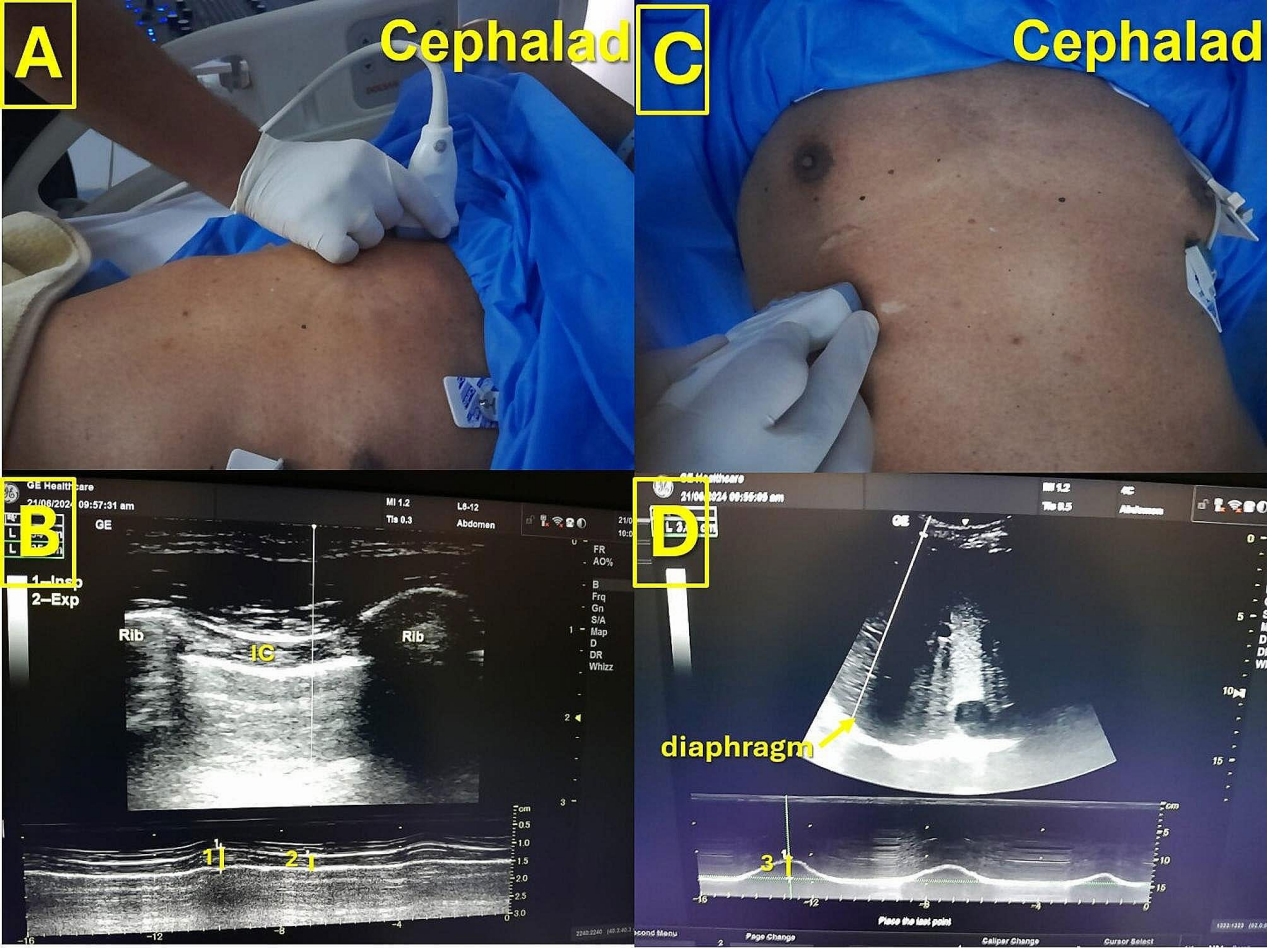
Ultrasound assessment of respiratory muscle. A: Transducer placement for assessment of parasternal intercostal thickening fraction. B: Inspiratory (yellow line 1) and expiratory (yellow line 2) intercoastal muscle thickness were measured using the M-mode. C: Transducer placement for assessment of diaphragmatic excursion. D: The amplitude of diaphragmatic muscle (yellow arrow) excursion between inspiration and expiation (yellow line 3) was then measured using the M-mode. IC: intercostal muscle
